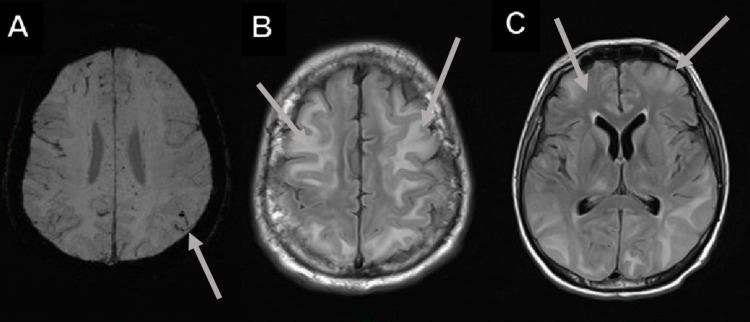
White matter BMB and images with suspected leukoencephalopathy. (A) SWI image with multiple juxtacortical and white matter BMB. (B and C) Subcortical bilateral asymmetrical hyperintensities in the T2-FLAIR images; suspected leukoencephalopathy. BMB: brain microbleeds; T2-FLAIR: T2-weighted fluid-attenuated inversion recovery
